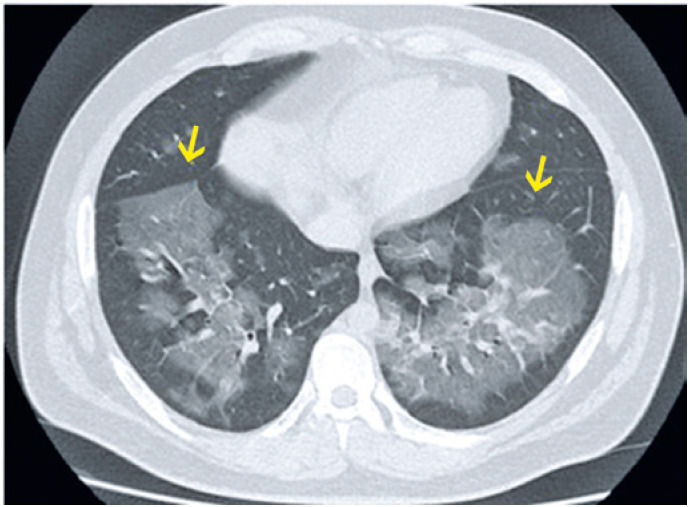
A 24-year-old male patient presented with dyspnea and cough. He reported the use of vaping. Computed tomography (axial): ground-glass opacities with diffuse peribronchovascular distribution, with predominance in the pulmonary bases, associated with thickening of the bronchial walls and sometimes thickening of the interlobular septa, in addition to small areas of consolidation in the bases
